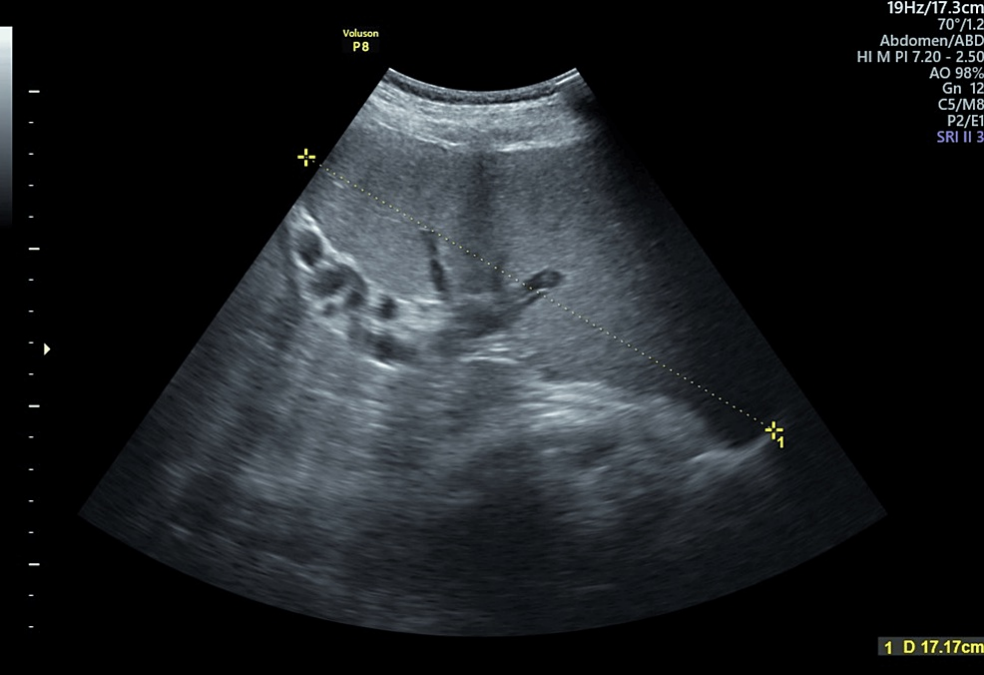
Ultrasonography revealing the presence of splenomegaly Yellow line indicates the length of the spleen
